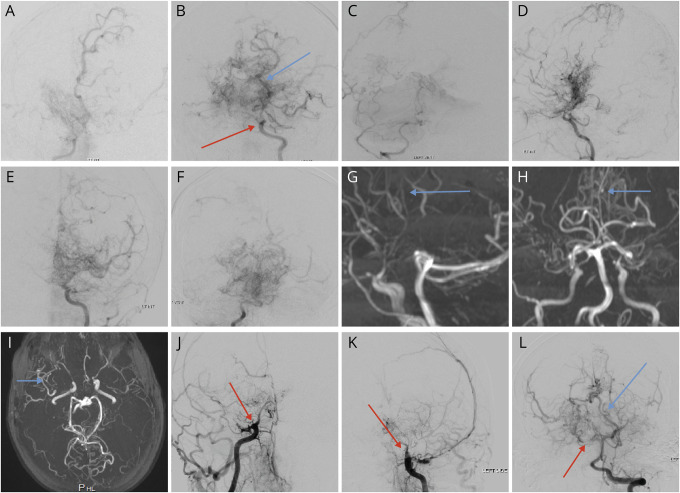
Cerebral and MRI angiography findings in patients with heterozygous CBL mutations and cerebral arteriopathy (A–C) Catheter angiogram (CA) for B-III-2 demonstrated a small and poorly filling right internal carotid artery (RICA), stenosed at the skull base (red arrow) and occluded at cavernous segment. The hemisphere was filled via moyamoya collaterals (red arrow) and a fetal right posterior cerebral artery (PCA). The left terminal internal carotid was severely stenosed and filled only a stenosed left middle cerebral artery (MCA) and multiple moyamoya collaterals. The right vertebral artery (VA) and basilar artery were small and did not fill the PCAs. (D–F) CA images for B-III-1 are shown. The RICA was occluded its cavernous segment with filling of some vessels via multiple moyamoya collaterals. The left ICA was occluded at the cavernous segment with severe stenosis of proximal anterior cerebral artery (ACA) and MCA and multiple moyamoya collaterals. The left VA was occluded, and the right VA is stenosed shortly before forming the basilar artery, which is small and which was occluded at it terminus with no filling of the PCAs. Multiple pial collaterals were visible. (G–I) (blue arrows) MRA images for B-II-2 revealed bilateral occlusion of the terminal ICAs (red arrows) with filling of distal vessels via moyamoya collaterals. The posterior circulation appeared patent but attenuated with pial collateral formation. (J–L) CA for C-II-6 revealed bilateral occlusion of the terminal ICAs with no distal filling via normal intracranial vasculature but some filling of the hemispheres from external carotid–internal carotid and skull base collaterals. The P1 segment of the right PCA was occluded (red arrow), but multiple pial collaterals were seen filling the hemispheres (blue arrow). CBL = casitas B-lineage lymphoma.
